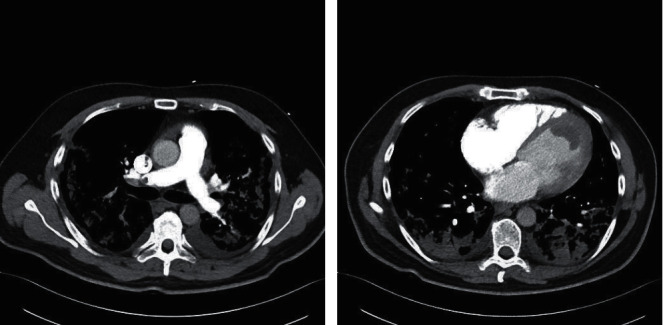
CT pulmonary angiogram. (a) Evidence of pulmonary thrombi at the bifurcations of the right main pulmonary artery and (b) cardiomegaly with an LV filling defect suggestive of a thrombus and multiple areas of consolidation consistent with SARS-CoV-2 infection.
